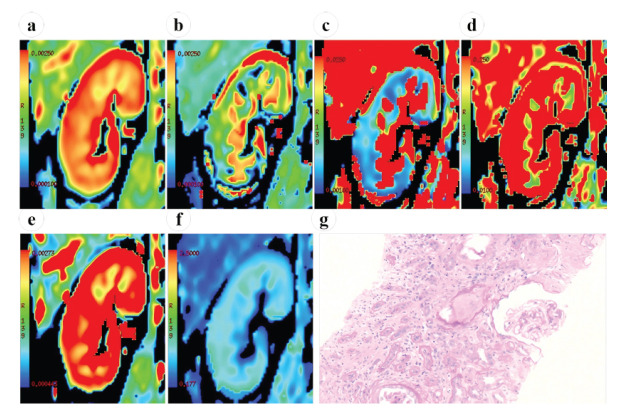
Magnetic resonance images in the coronal plane and corresponding pathological changes in a 45-year-old man with CKD and a low eGFR (66.3 mL/min/1.73 m^2^). (a) ADC map (ADC: 2.15 × 10^-3^ mm^2^/s). (b) ADC_slow_ map (ADC_slow_: 1.65 × 10^-3^ mm^2^/s). (c) ADC_fast_ map (ADC_fast_: 15.6 × 10^-3^mm^2^/s). (d)
